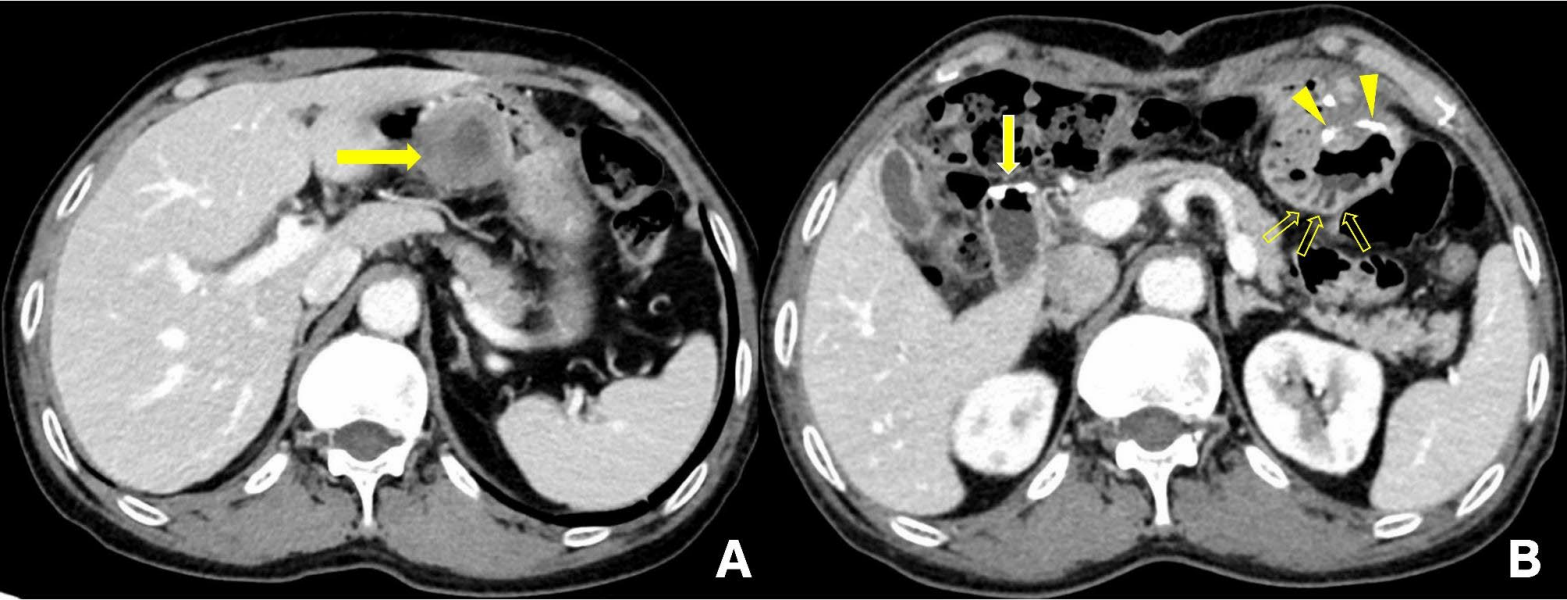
Contrast-enhanced abdominal computed tomography images(case 6). A: Preoperative CT imaging (December 2019): CT scan shows a large, exophytic mass with heterogeneous enhancement in the wall of the distal stomach. B: Postoperative CT imaging following Billroth II gastrojejunostomy (September 2021): CT scan shows surgical staples at the gastrojejunal anastomosis site(arrowheads), where no local tumor recurrence was observed. The jejunal loop(open arrows) characterized by the valvulae conniventes is also noted. Metallic suture material(solid arrow) clearly indicates the duodenal stump
